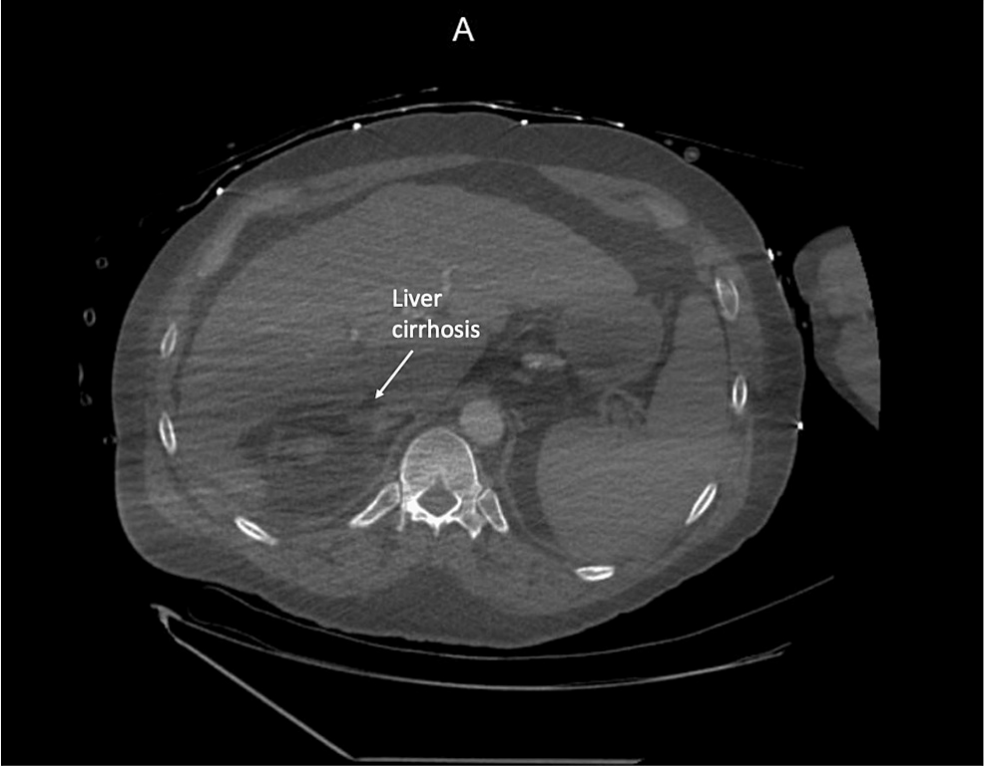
CT scan of the abdomen and pelvis shows signs of liver cirrhosis with portal hypertension
